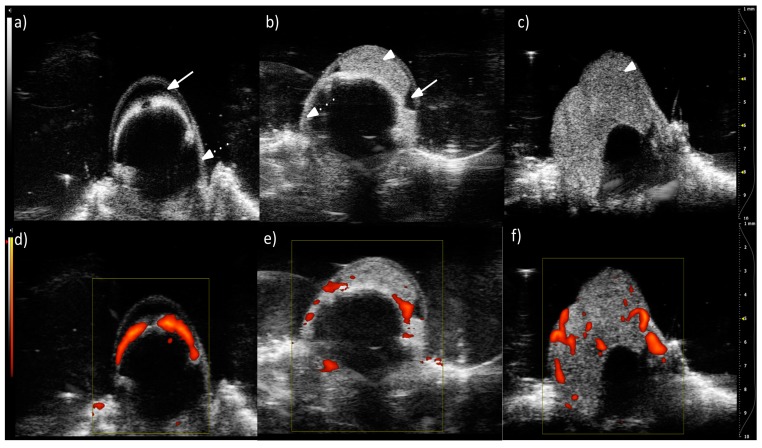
Single cross-section from 3D US images of the tumor in the eye of a living hamster. B-mode images showing a control eyeball (a); eyeball with a medium-sized tumor (b); and large tumor (c). Visible structures of the eye are indicated: anterior chamber (solid arrow), choroid (dotted arrow), tumor mass (arrowhead). In the lower panel, the Doppler mode is presented, showing vascular blood flow (color) in a control eyeball (d); eyeball with a medium-sized tumor (e); and large tumor (f). All colored areas correspond to blood flow. Darker colors indicate lower frequency signals. Scale on the right is in mm.
